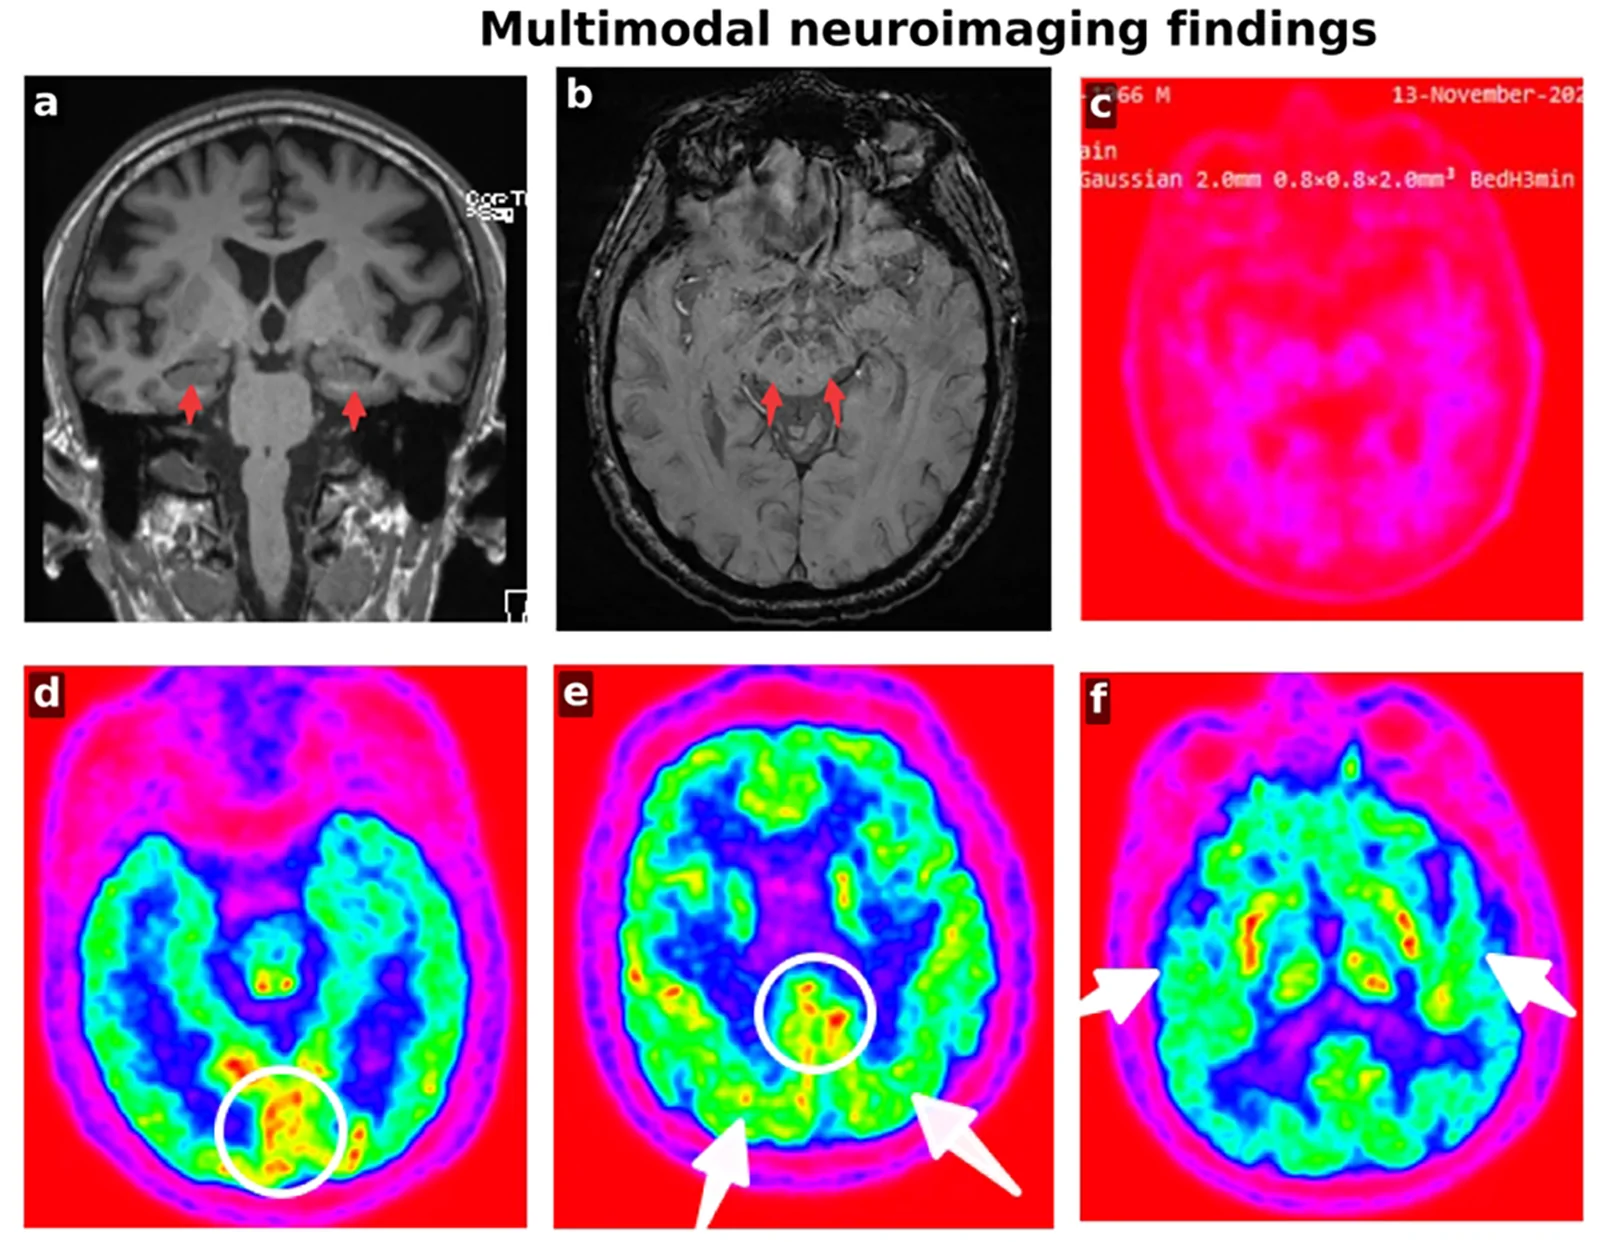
Multimodal neuroimaging findings. (A) Brain MRI showing medial temporal atrophy (score 1). (B) SWI demonstrating bilateral nigrosome-1 signal loss (“swallow tail sign” absent). (C) Amyloid-beta PET ([18F]florbetapir) showing no cortical tracer retention. (D) FDG-PET showing relative preservation of primary visual cortex (V1) (white circle). (E) Cingulate island sign (white circle: preserved posterior cingulate cortex metabolism; white arrows: bilateral occipital hypometabolism). (F) Lateral temporal hypometabolism (white arrows).
